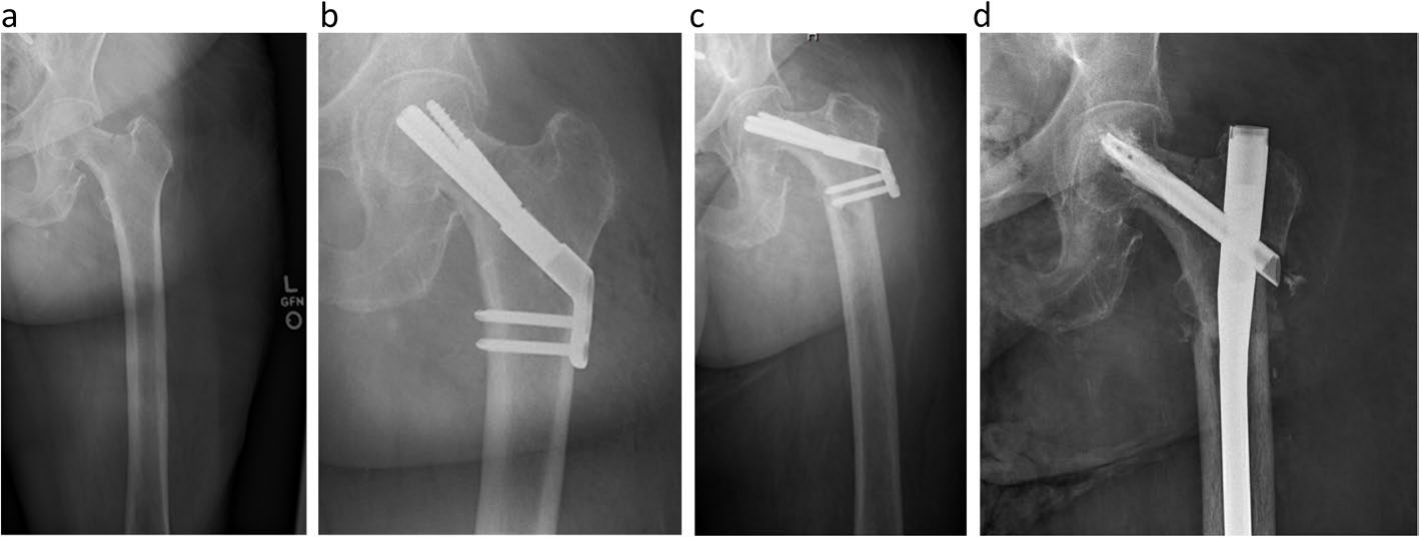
68-year-old female with 1 week of progressive hip pain. Panel a is the injury AP radiograph of the left hip showing an incomplete non-displaced fracture. Panel b are postoperative AP radiographs of the left hip showing the implanted femoral neck system. Panel c is an AP radiograph of the left hip taken 41 days postoperatively revealing a peri-implant subtrochanteric fracture. Panel d is an AP radiograph of the left hip taken at 6-week postoperative visit showing successful treatment with a cephalomedullary nail
